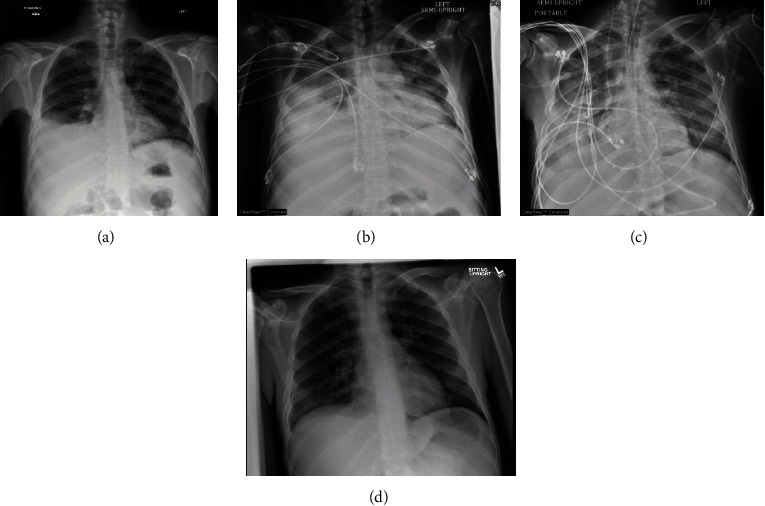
Serial chest X-rays (CXR) showing progression of parenchymal opacities consistent with ARDS. (a) Day of admission, with mild parenchymal opacities at the R lung base. (b) Hospital day 4, with worsening bilateral parenchymal opacities. (c) Hospital day 6, with ongoing bilateral parenchymal opacities. (d) Hospital day 45, parenchymal opacities have resolved.
